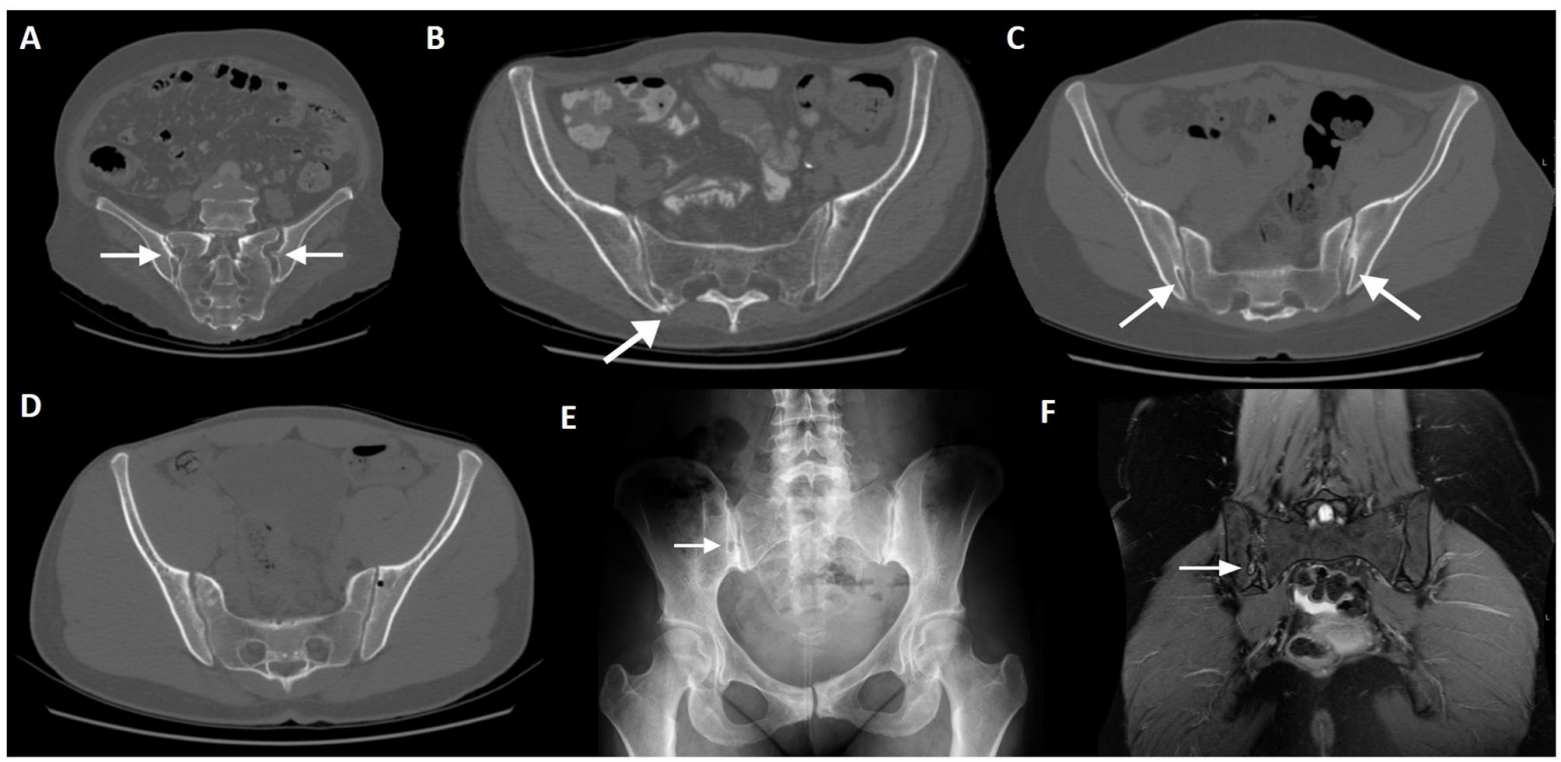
Normal variants and incidental findings of the sacroiliac joint (arrows). CT reconstructions with oblique orientation depict bilateral iliosacral complexes (A), the most common sacroiliac joint variant; right accessory sacroiliac joint (B); bilateral bipartite iliac bony plate (C); left iliac bone pneumatocyst (D). A patient with an incidental finding on the right sacroiliac joint seen on pelvic radiography performed MRI, which revealed an iliac bone cleft filled with fluid (E,F). Note the sclerosis of the symphysis pubis (E), compatible with *osteitis pubis*.
